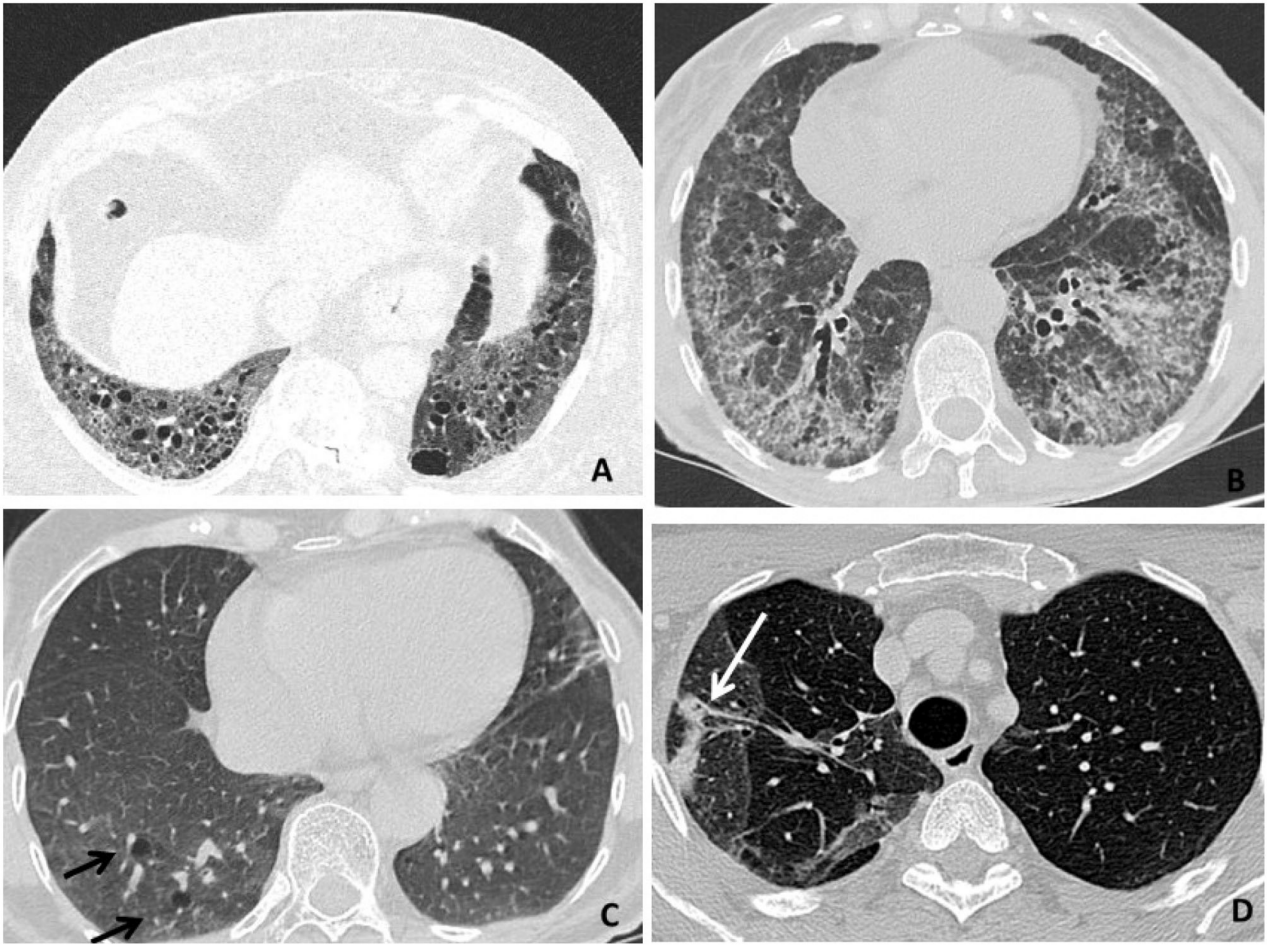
High-resolution computer tomography (HRCT) findings in respiratory diseases and particularly interstitial disease (RA-ILD). (A) An usual interstitial pneumonia (UIP) pattern (representing the most typical radiological presentation in patients with RA) is primarily characterized by reticular fibrosis, traction BR, and subpleural cysts (honeycombing). (B) A nonspecific interstitial pneumonia (NSIP) pattern is primarily characterized by ground-glass opacities, variably mixed with septal thickening, and reticular fibrosis (fibrosing NSIP). (C) A lymphocytic interstitial pneumonia (LIP) pattern is primarily characterized by perivascular thin-walled cysts (black arrows). (D) An organizing pneumonia (OP) pattern is primarily characterized by pulmonary consolidations (a white arrow).
